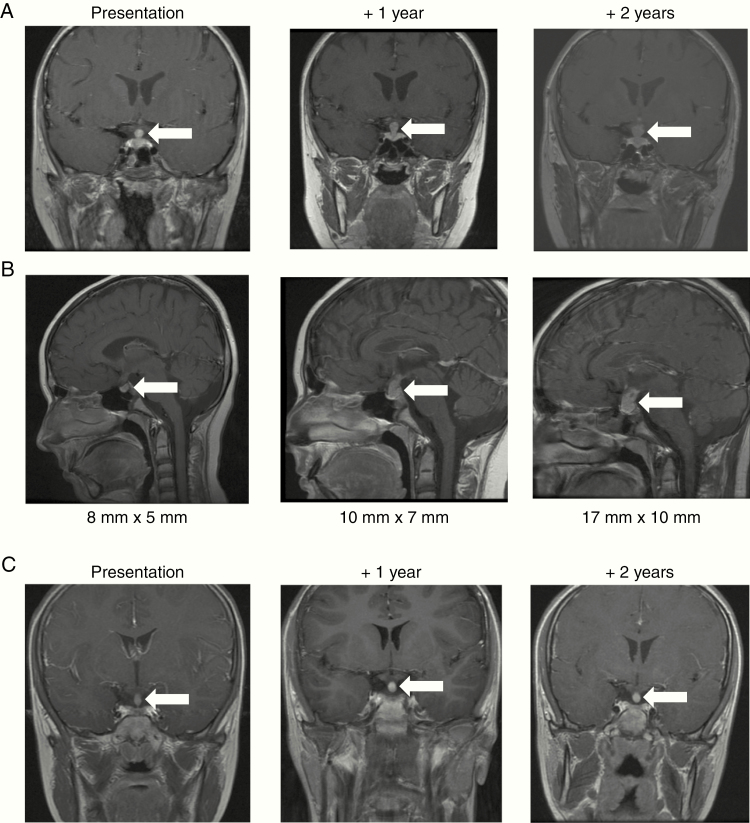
MRI of Cases 2 and 3 presenting with pituitary thickening and diabetes insipidus. Representative (A) coronal views of Case 2, (B) sagittal views of Case 2, and (C) coronal views of Case 3—at first presentation (left panels), 1 year later (central panels), and 2 years later (right panels). White arrows indicate the radiological abnormality.
